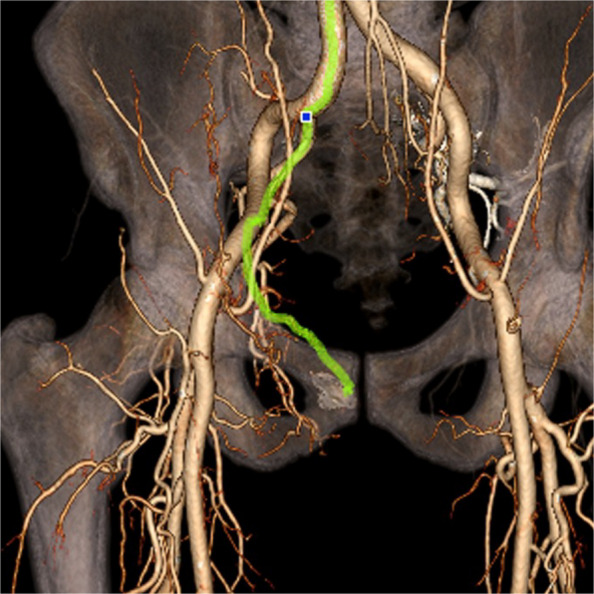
CTA reformat, planning for fusion
